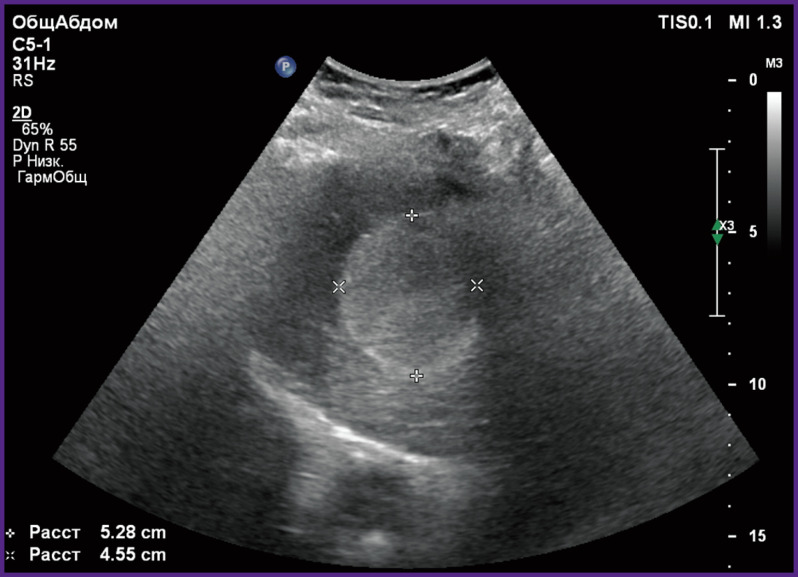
Patient L., 42 years old. Echosonogram of hepatic hemangioma
